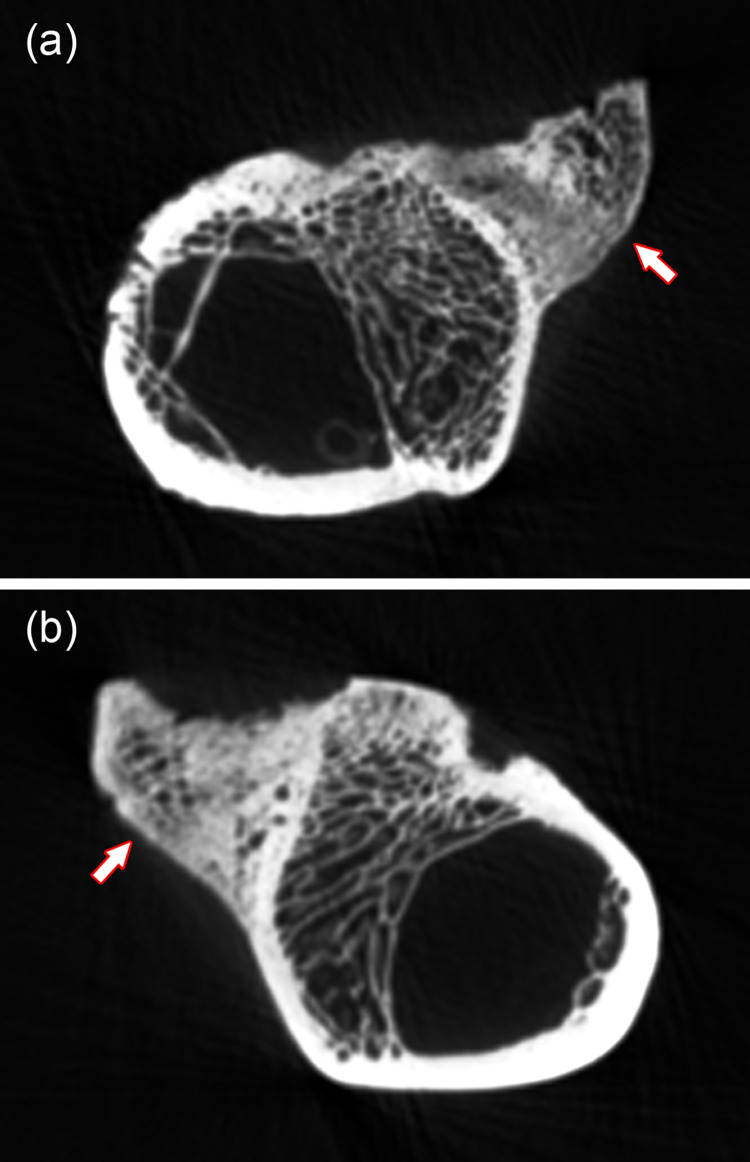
CT-images (transverse slices) of the pathological femora (proximal portion, below femoral head). (a) Right femur. (b) Left femur. Arrows: Bony outgrowths in the region of the intertrochanteric crest.
